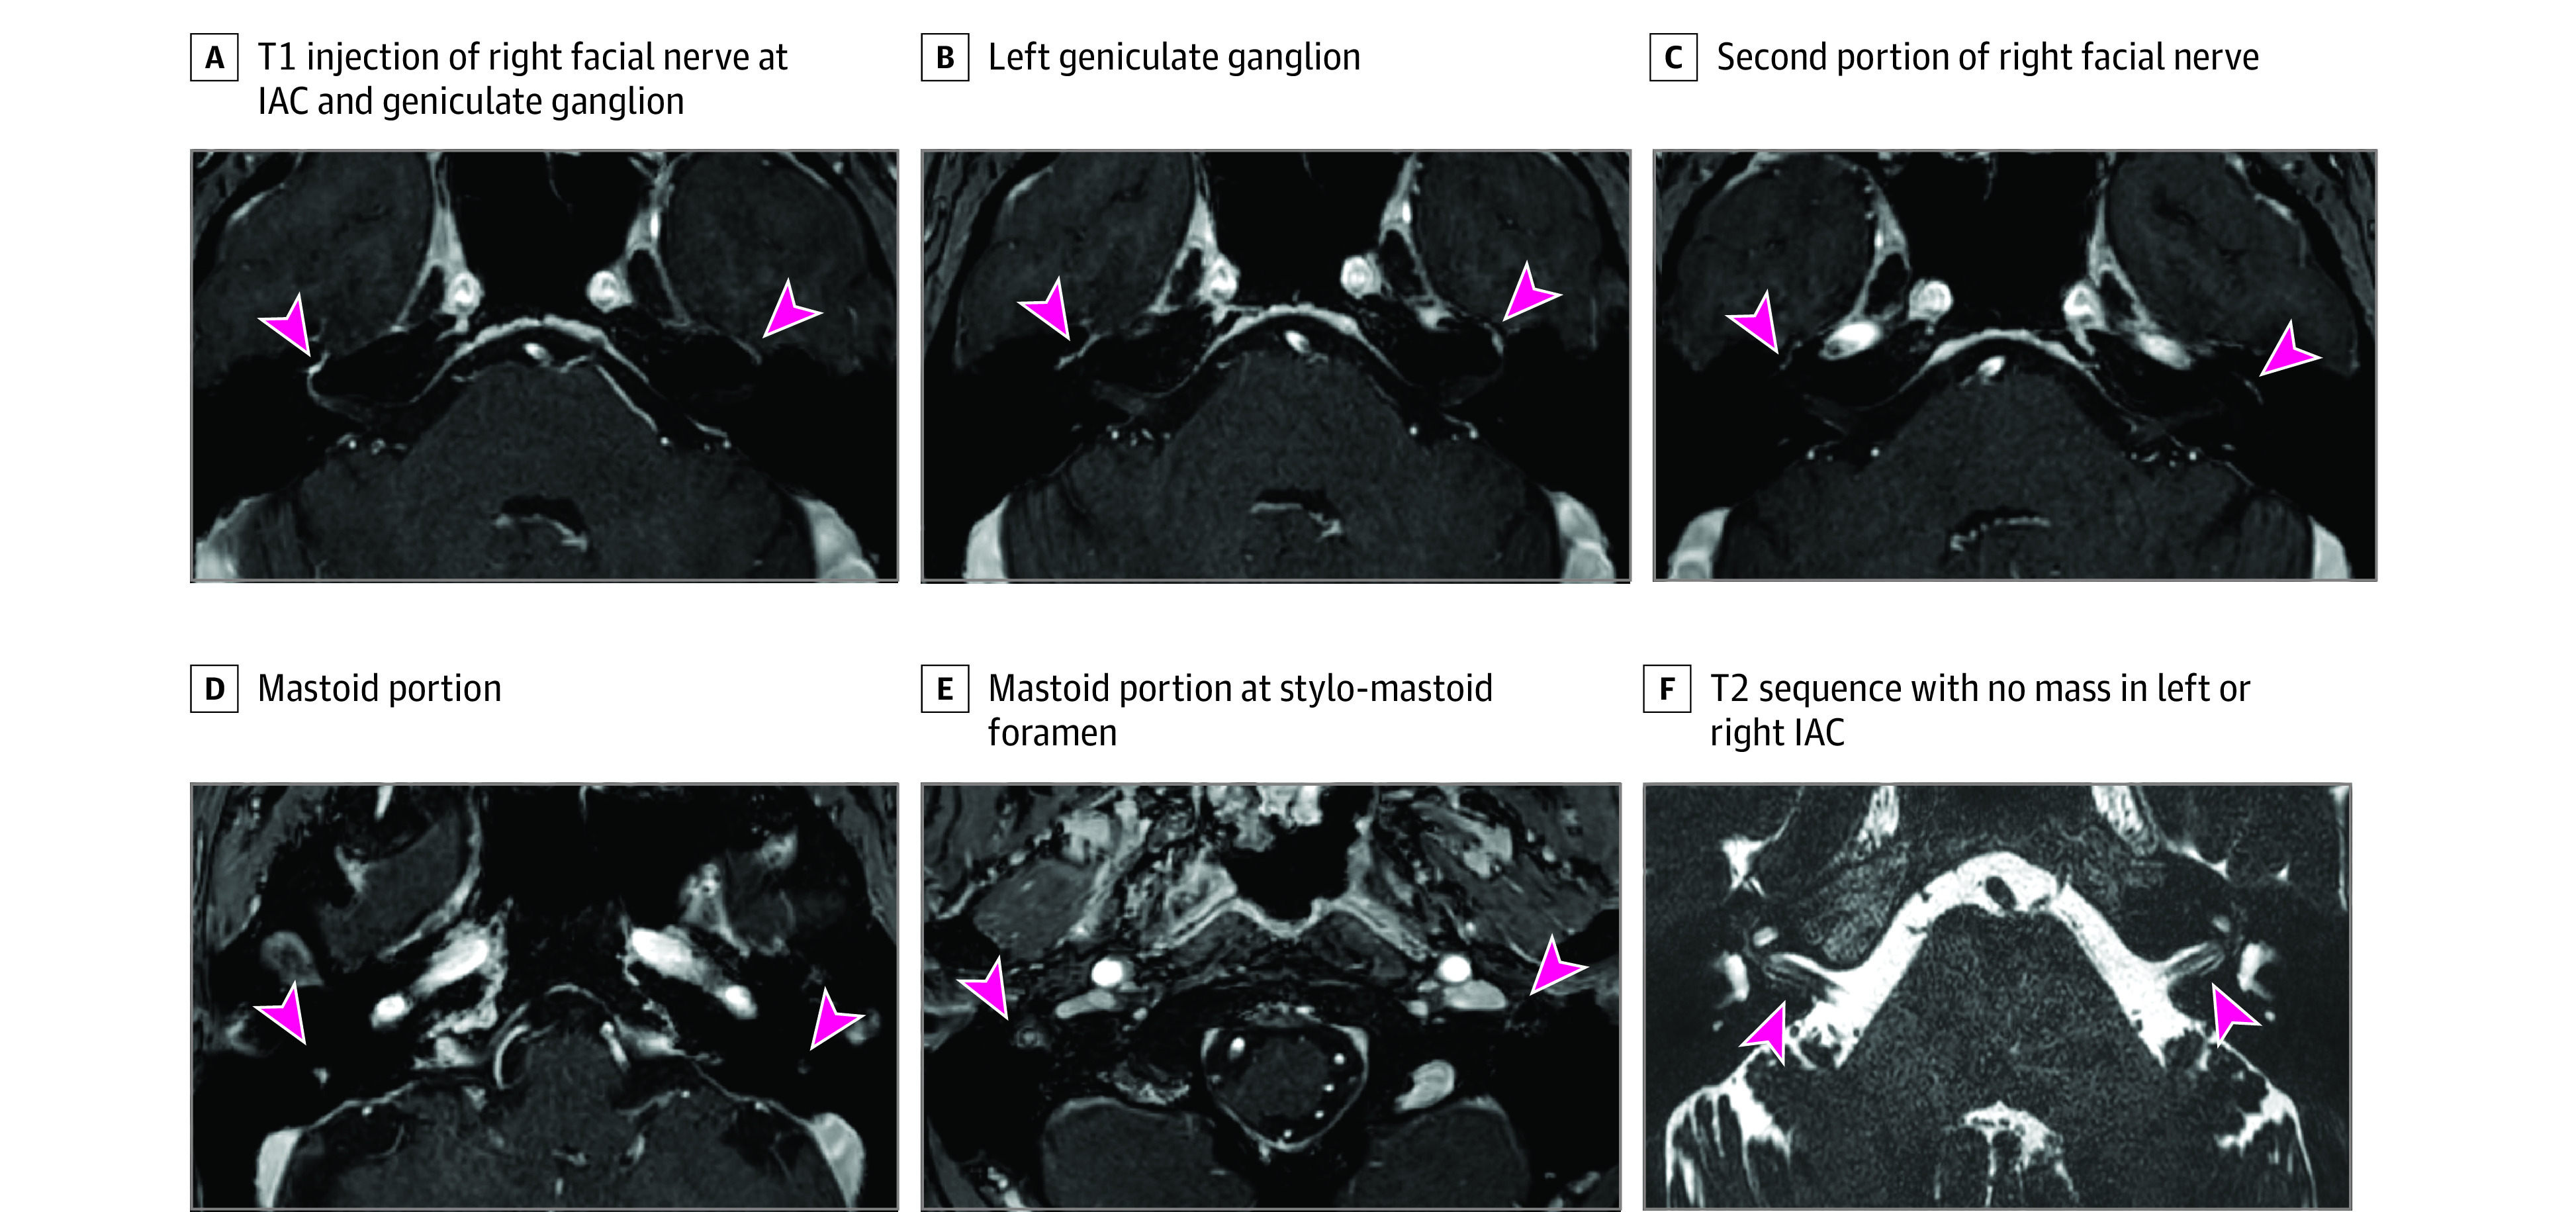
Magnetic Resonance Images of a Patient in Their 40s With Right Idiopathic Peripheral Facial Palsy Images were obtained 5 weeks after presentation to the emergency department, showing typical signs of right facial nerve neuritis. Left arrowheads indicate increased hyperintensity after gadolinium injection; right arrowheads indicate the left facial nerve for comparison. IAC indicates internal auditory canal.
